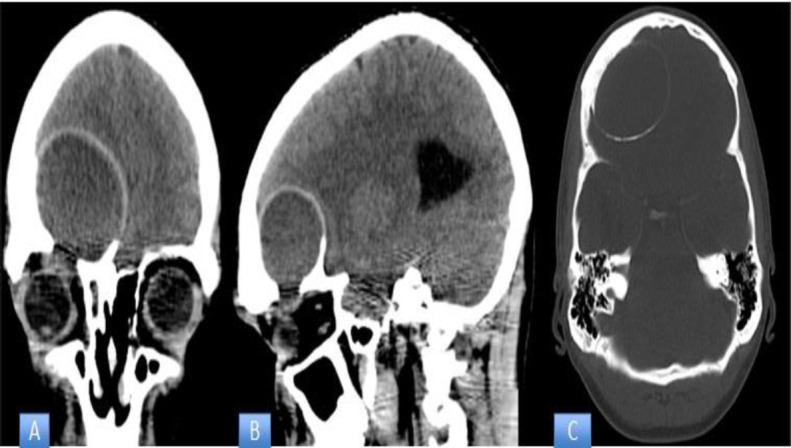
CT scan in a coronal, B sagittal and C axial planes. Mucopyocele of the roof of the right orbit, with calcified walls and extension to the anterior cranial fossa displacing the adjacent brain tissue. The area of the mucopyocele is indicated with a black arrow
